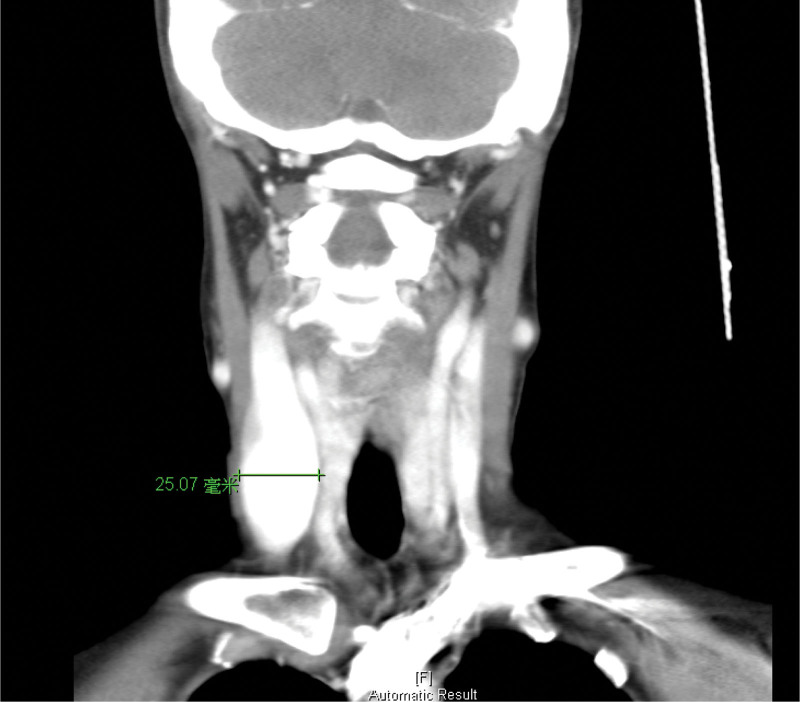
Coronal view of head and neck CT showed a right fusiform internal jugular vein aneurysm, 25.07 mm in maximum width. There was a filling defect, which was a thrombus. CT = computed tomography.
